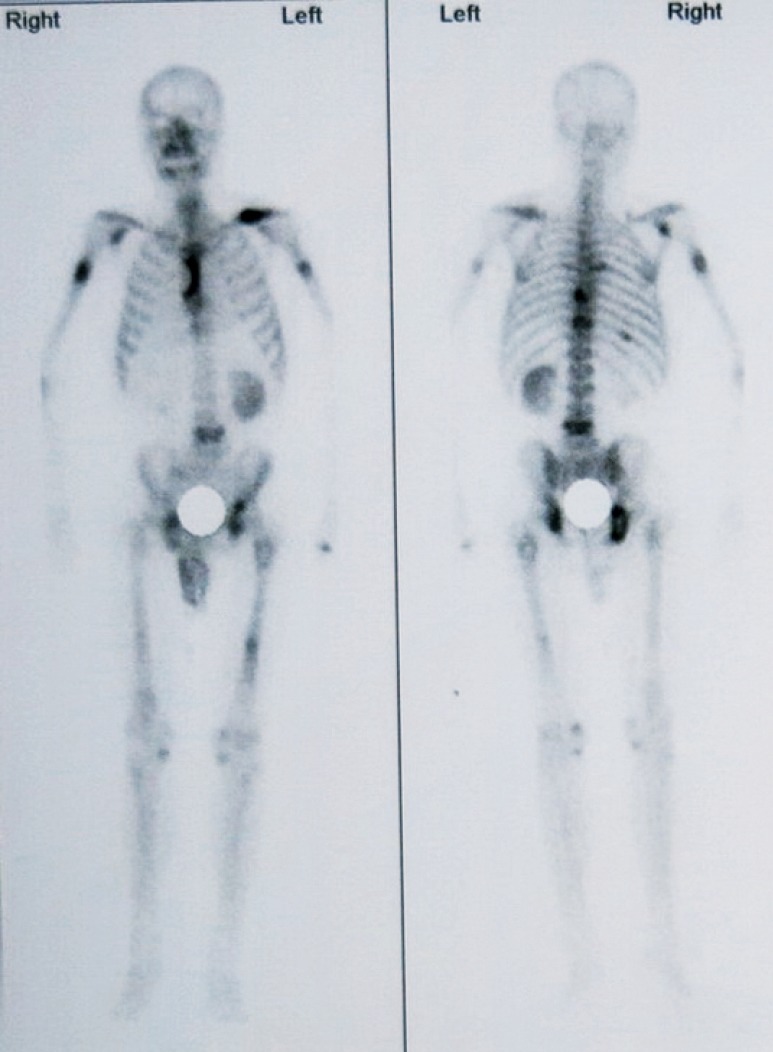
Technetium-99m–methylene diphosphonate bone scintigraphy revealed multiple radioactive foci.
